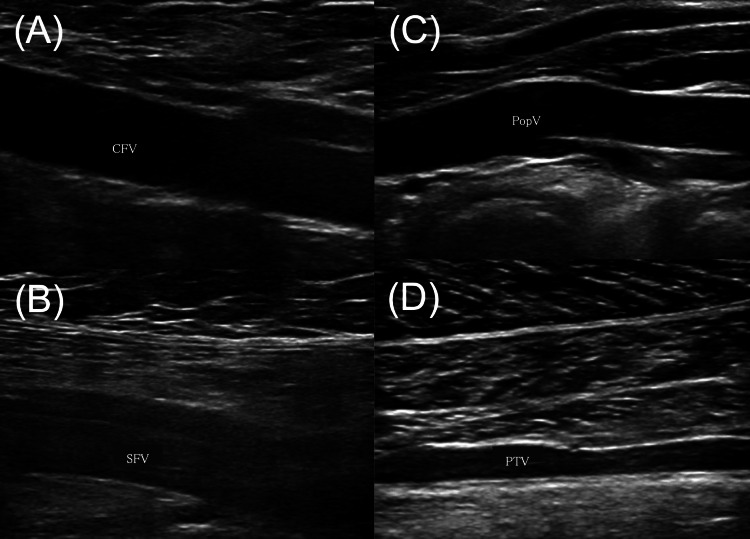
Lower-extremity venous ultrasound images before fracture surgery, demonstrating no deep vein thrombosis (A) Common femoral vein; (B) Superficial femoral vein; (C) Popliteal vein; (D) Posterior tibial vein
